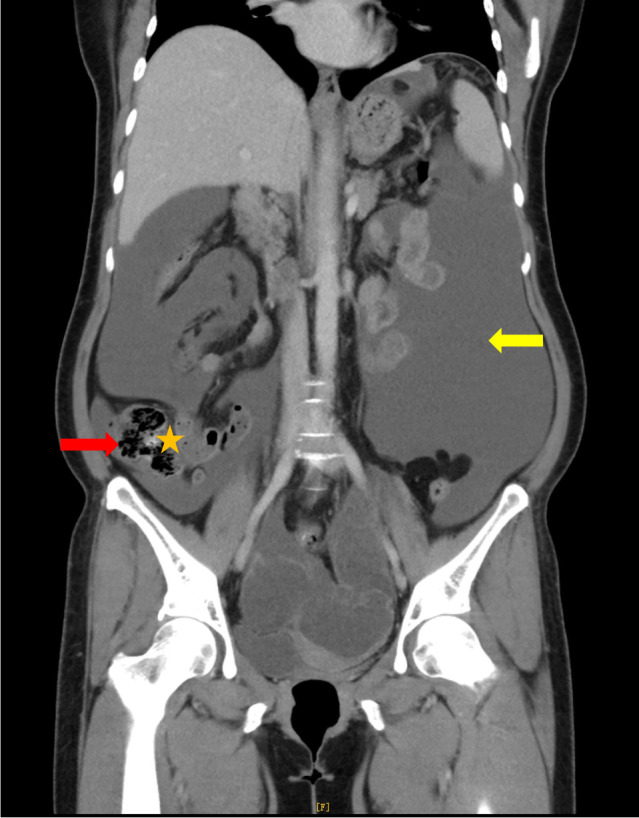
Preoperative contrast-enhanced CT of abdomen-pelvis-chest (coronal view). It demonstrates massive, partially loculated ascites filling the abdominal cavity (yellow arrow), alongside giant bilateral multiloculated cystic adnexal masses (red arrow) with punctate calcification on the cyst wall (orange star).
